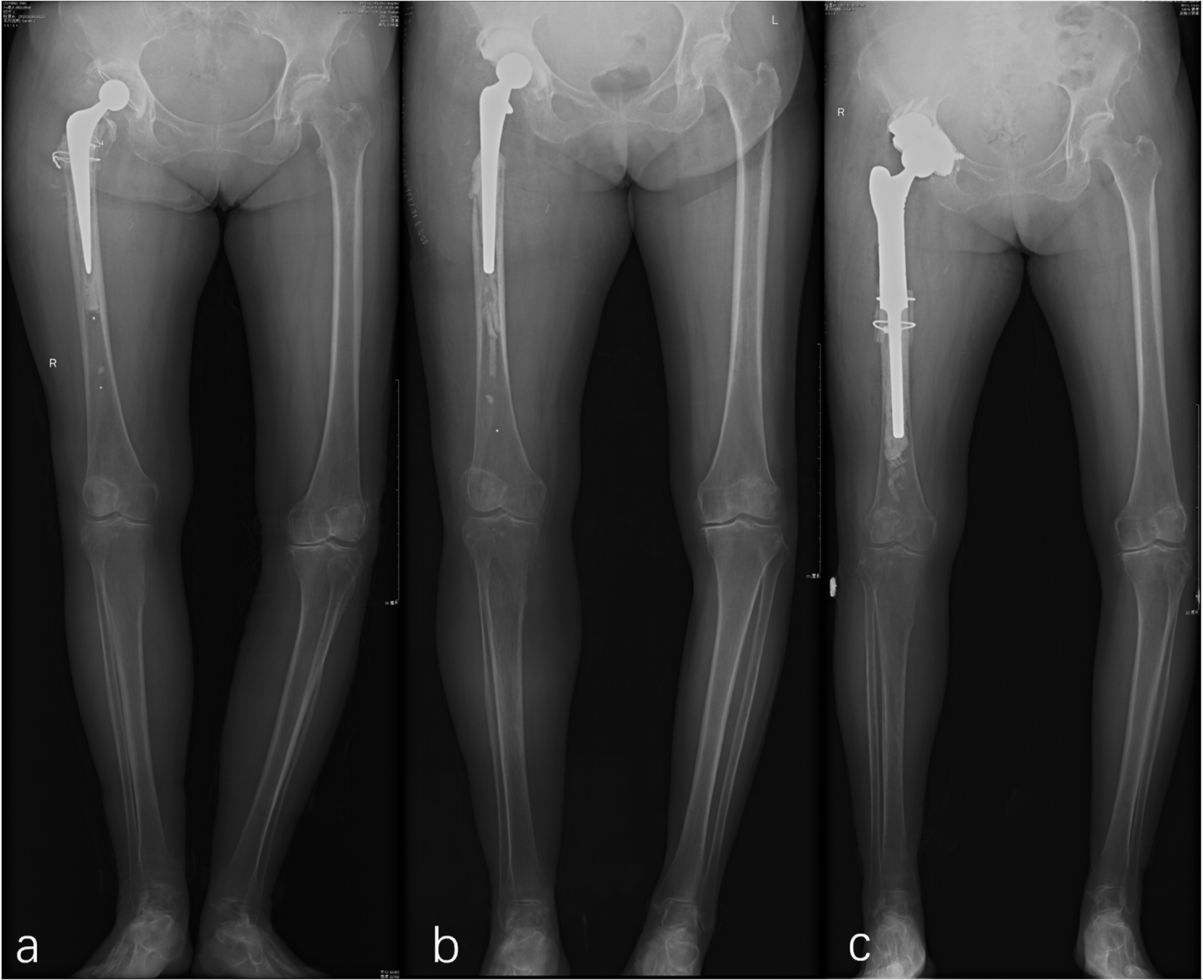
X-ray films of this two-stage treatment. a: X-ray film of the patient before the first stage operation; b: X-ray film of the patient after the first stage operation; c: X-ray film of the patient before the second stage operation
